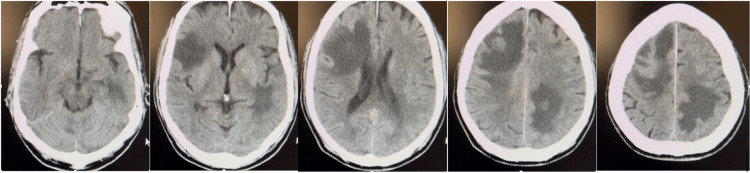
CT head images from left to right show multifocal intracranial lesions with vasogenic edema indicative of metastases, proceeding in a caudocephalad direction.
